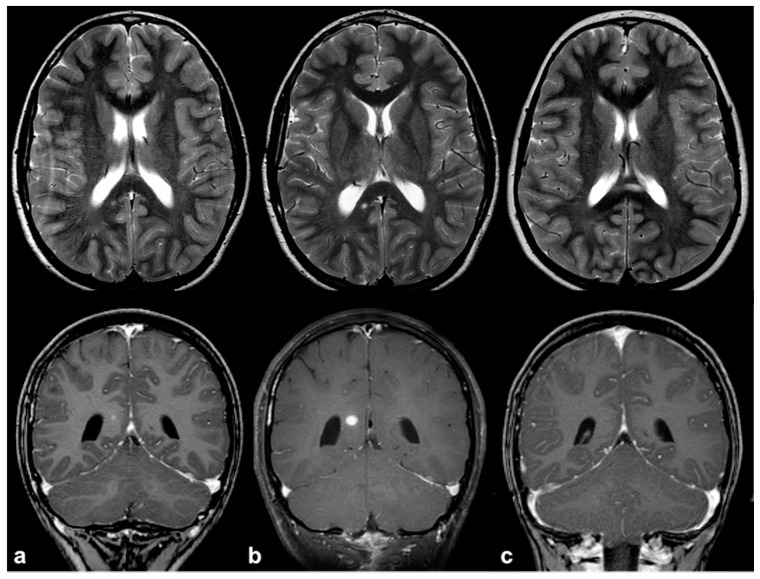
Longitudinal follow-up of atypical brain FASI in a 5 year-old NF1 male patient: axial TSE T2w (first row) and coronal post-contrast T1w (second row) MRI showing a oval-shaped lesion into the right occipital forceps of the splenium corpus callosum at three different time points (a = 5, b = 5.5, and c = 6 years old). The lesion was T2-hyperintense with punctate enhancement at diagnosis, showed a transient volume increase with focal contrast enhancement, and then completely regressed at one-year longitudinal MRI follow-up.
